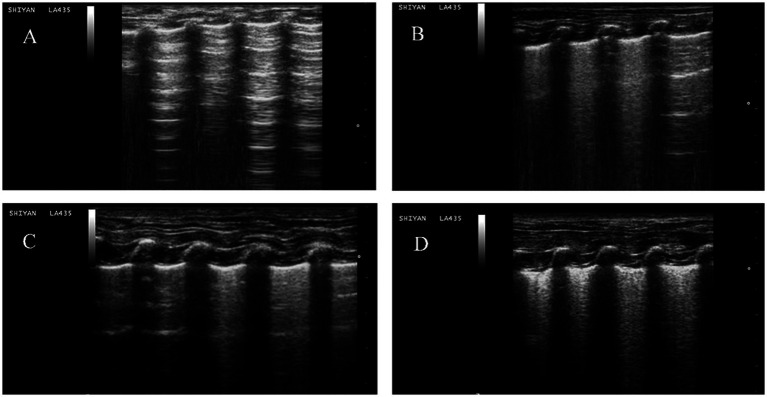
Representative lung ultrasound images for each group. (A) NC group, A-lines accompanying pleural sliding. (B) OM group, multiple spaced B-lines, with local A-lines. (C) LOM group, multiple spaced B-lines, with local partially coalescent B-lines. (D) LOV group, multiple coalescent B lines, substantial echogenic areas with hyperechoic dot-like patterns, and static or dynamic signs of bronchial inflation.
